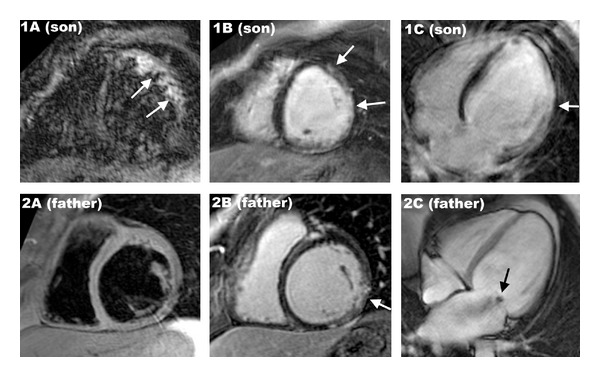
Panel A: son short-axis view T2 weight cardiac MRI with black blood and fat saturation showing bright signal intensity of the lateral wall (white arrows) suggesting myocardial oedema. Panels 1B and 1C: son short-axis view (1B) and 4-chamber view (1C) cardiac MRI showing subepicardial late gadolinium enhancement of the lateral wall (white arrows) suggesting diagnosis of myocarditis. Panel 2A: father short-axis view T2 weight cardiac MRI with black blood and fat saturation without signal abnormality. Panel 2B: father short-axis view (1B) cardiac MRI showing subepicardial late gadolinium enhancement of the inferolateral wall (white arrow) suggesting diagnosis of myocarditis. Panel 2C: father four-chamber view cine-MRI during the first part of the systole showing dilated cardiomyopathy with functional mitral regurgitation (black arrow).
